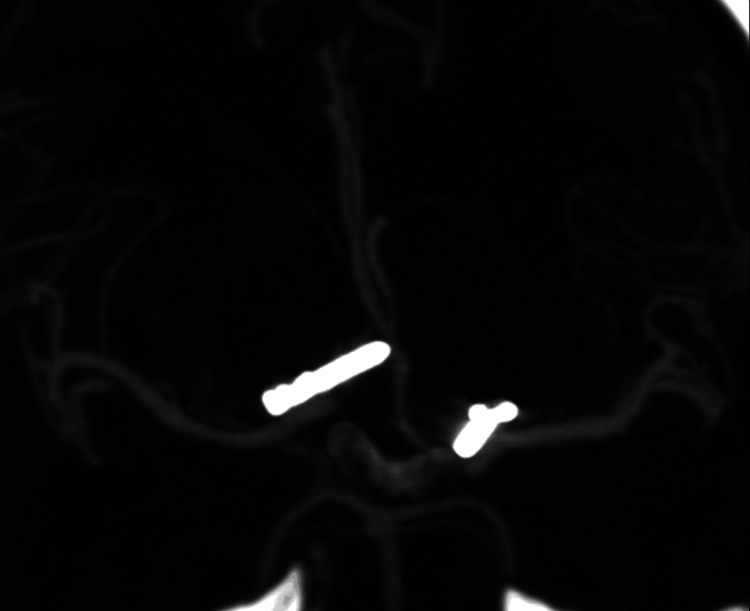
Computed tomographic angiography five days after initial clipping There was evidence of complete obliteration of both clipped aneurysms.
